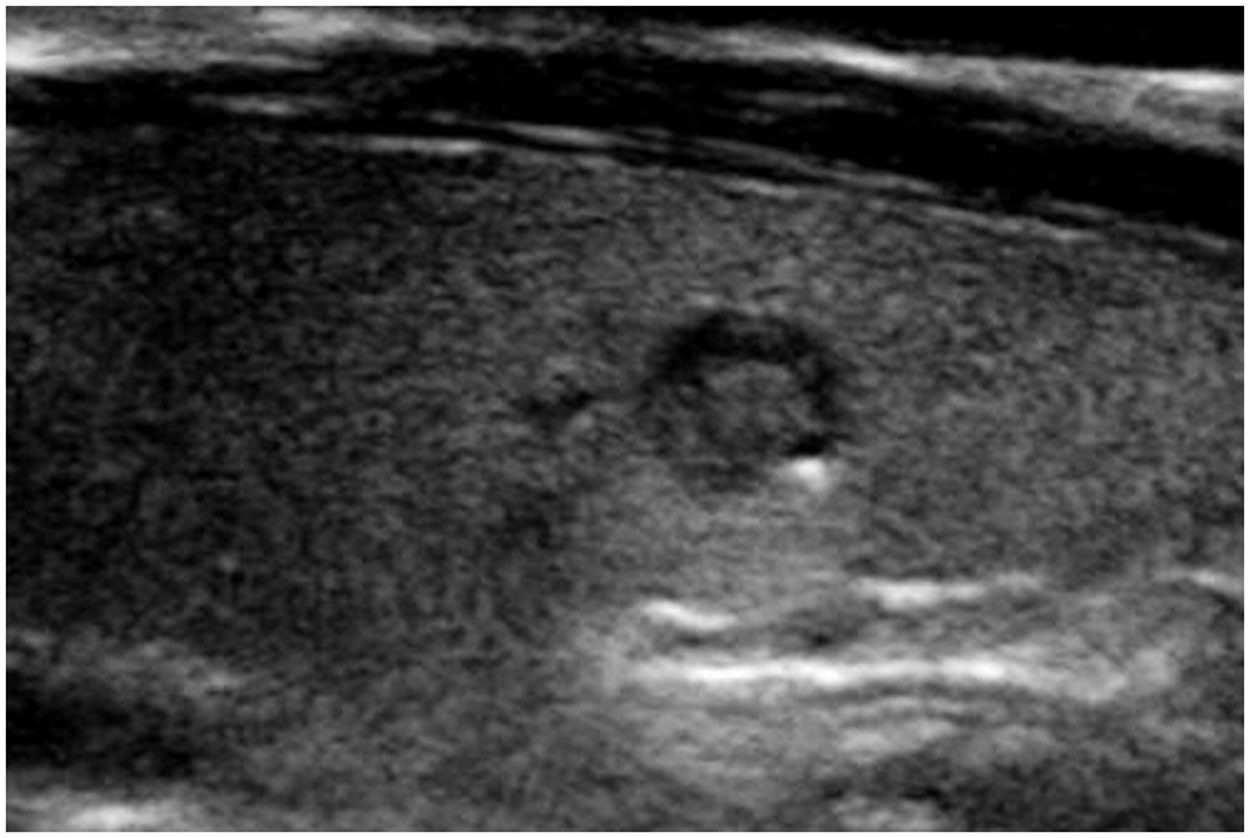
A 31-year-old woman with papillary thyroid carcinoma. Longitudinal sonogram shows a 5-mm, smooth, solid hypoechoic nodule in the right thyroid lobe. The echogenic focus (solid arrow) is located at the margin of intra-nodular micro-cyst. Comet-tail artifact was detected in the solid component (Type 2).
